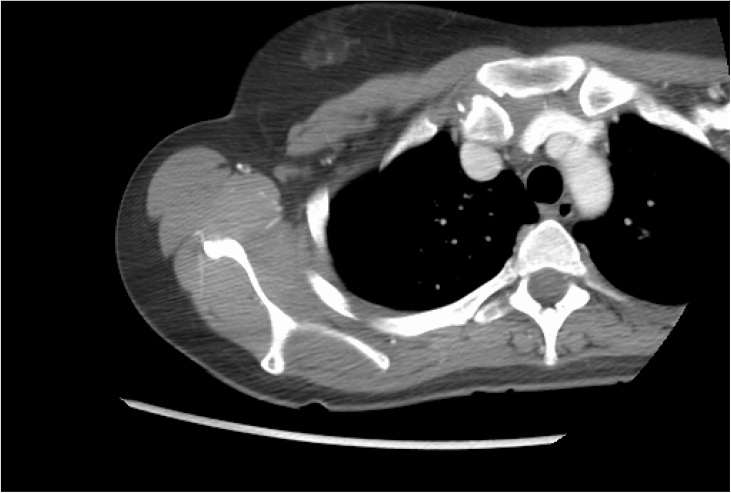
CT angiogram with the patients right arm above their head showed the medial end of the clavicle was posteriorly displaced as noted previously. There was no evidence of any vascular compression, either arterial or venous. The subclavian, axillary and brachial arteries were also normal.
